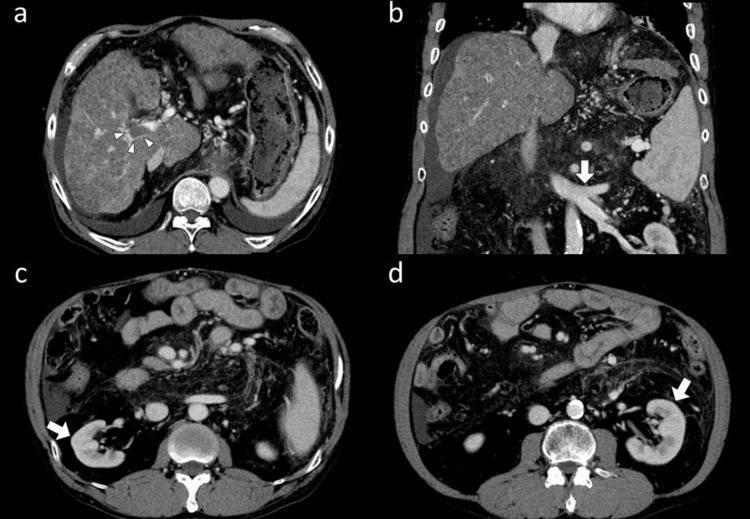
Pretransplant CT of the recipient who underwent DDLT Pretransplant CT findings of the recipient who underwent DDLT. a) The thrombus of the right portal vein was observed (arrowheads). b) Splenomegaly and splenorenal shunt (arrow) were shown. c and d) The bilateral kidneys were atrophic (arrows). Abbreviations: CT, computed tomography; DDLT, deceased donor liver transplantation
